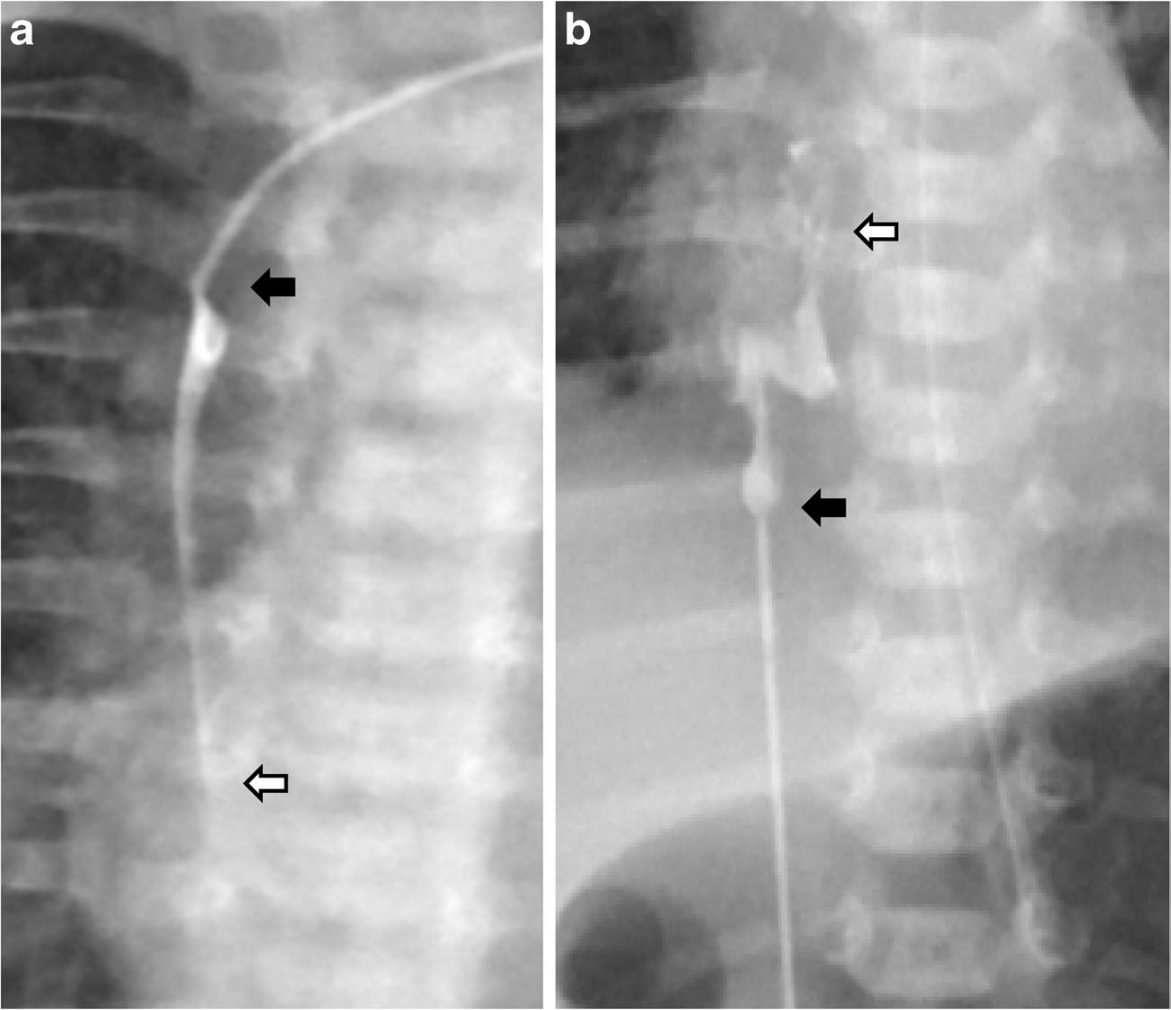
Radiographs show radiopaque agent jets (*white arrows*) beyond the peripherally inserted central catheter (PICC) tips (*black arrows*). a A 3-day-old girl. AP chest radiograph of upper limb PICC. b a 5-day-old boy. AP chest and abdomen radiograph of lower limb PICC
